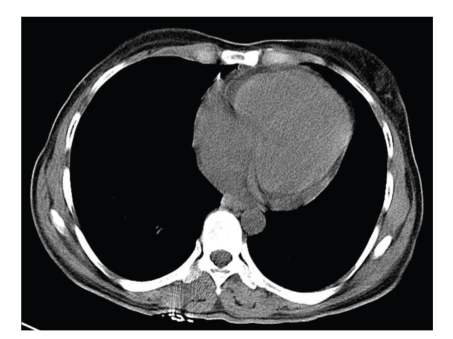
Computed Tomography scan (CT scan) of abdomen showed a minimal pericardial effusion.
